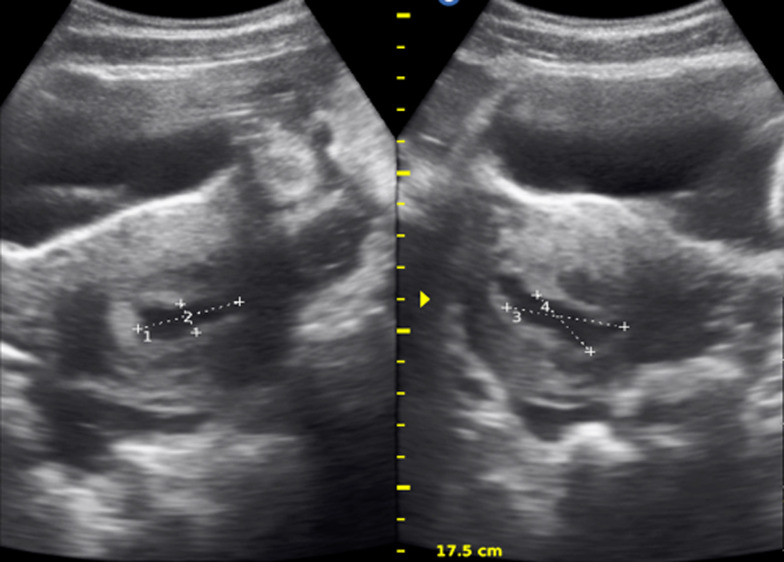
ultrasound image showing an intrauterine gestational sac at 7 weeks 2 days, free fluid at the pouch of Douglas
